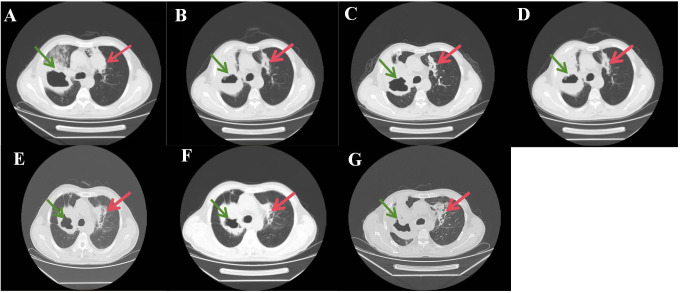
(A–G) track the primary right upper lobe cavitary lesion from pre-treatment baseline through 11 months of follow-up. Despite a transient radiographic flare in June 2025 (E) attributed to peritumoral inflammation, the solid tumor component remained overall stable consistent with Stable Disease (SD), and no new lesions appeared.
